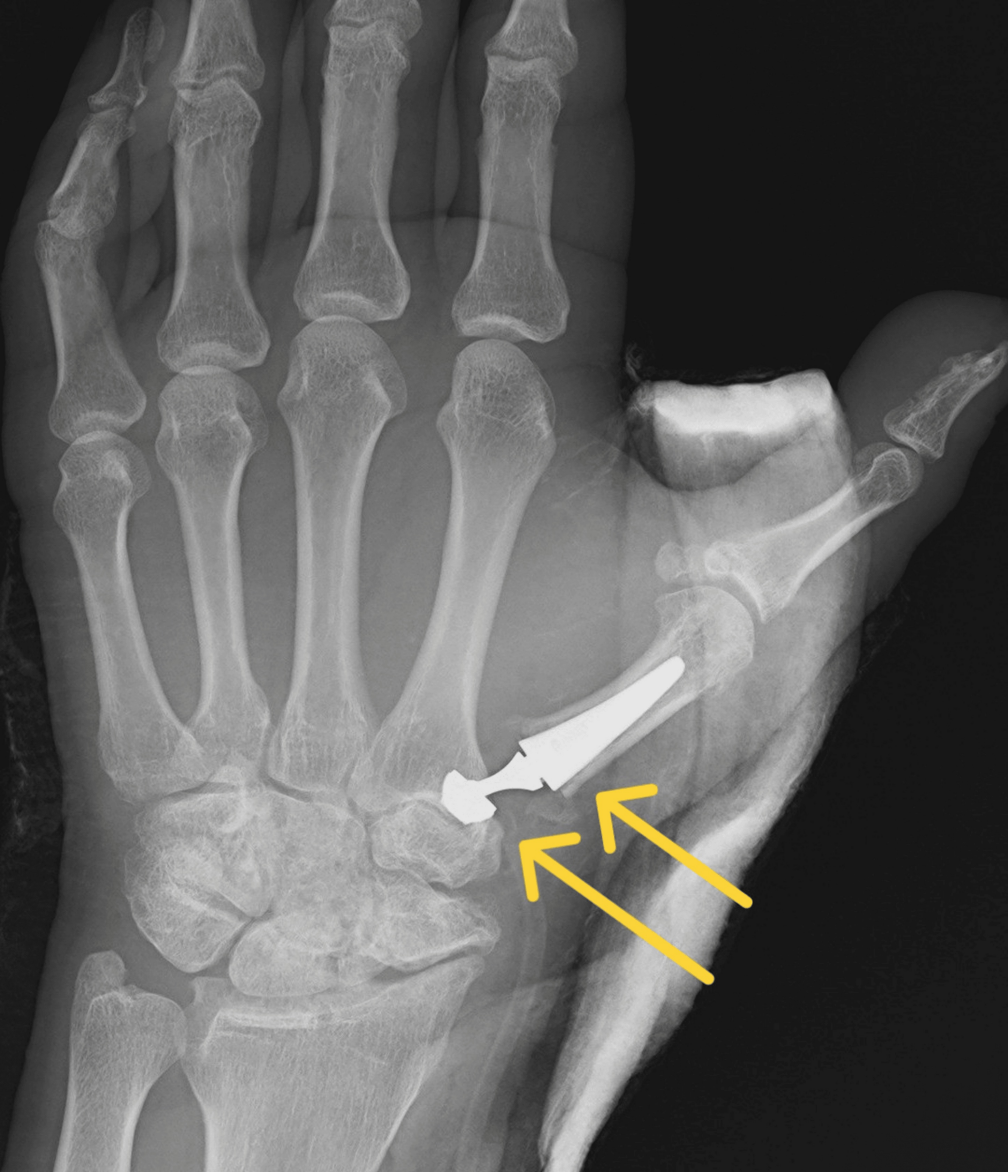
Control X-ray in A-P projection obtained after surgery A-P: anterior-posterior
